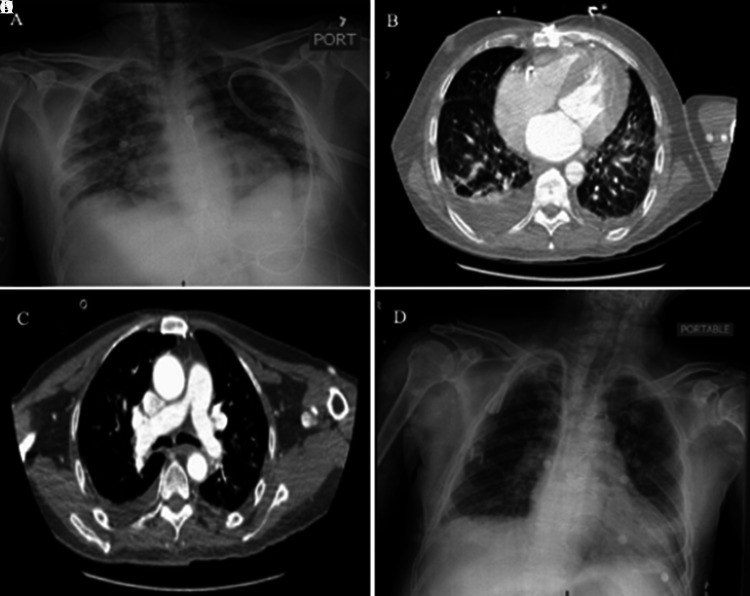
A: Chest X-ray (CXR) posteroanterior (PA) view of patient 1 showing patchy ground-glass opacities in all lung fields bilaterally. B: Chest CT-angiography of patient 2 showing diffuse ground glass opacities with micronodules and right-sided pulmonary effusion. C: Chest CT-angiography of patient 2 showing normal pulmonary arteries ruling out pulmonary embolism. D: CXR PA view of patient 3 showing diffuse, patchy ground-glass opacities in bilateral lung fields.
